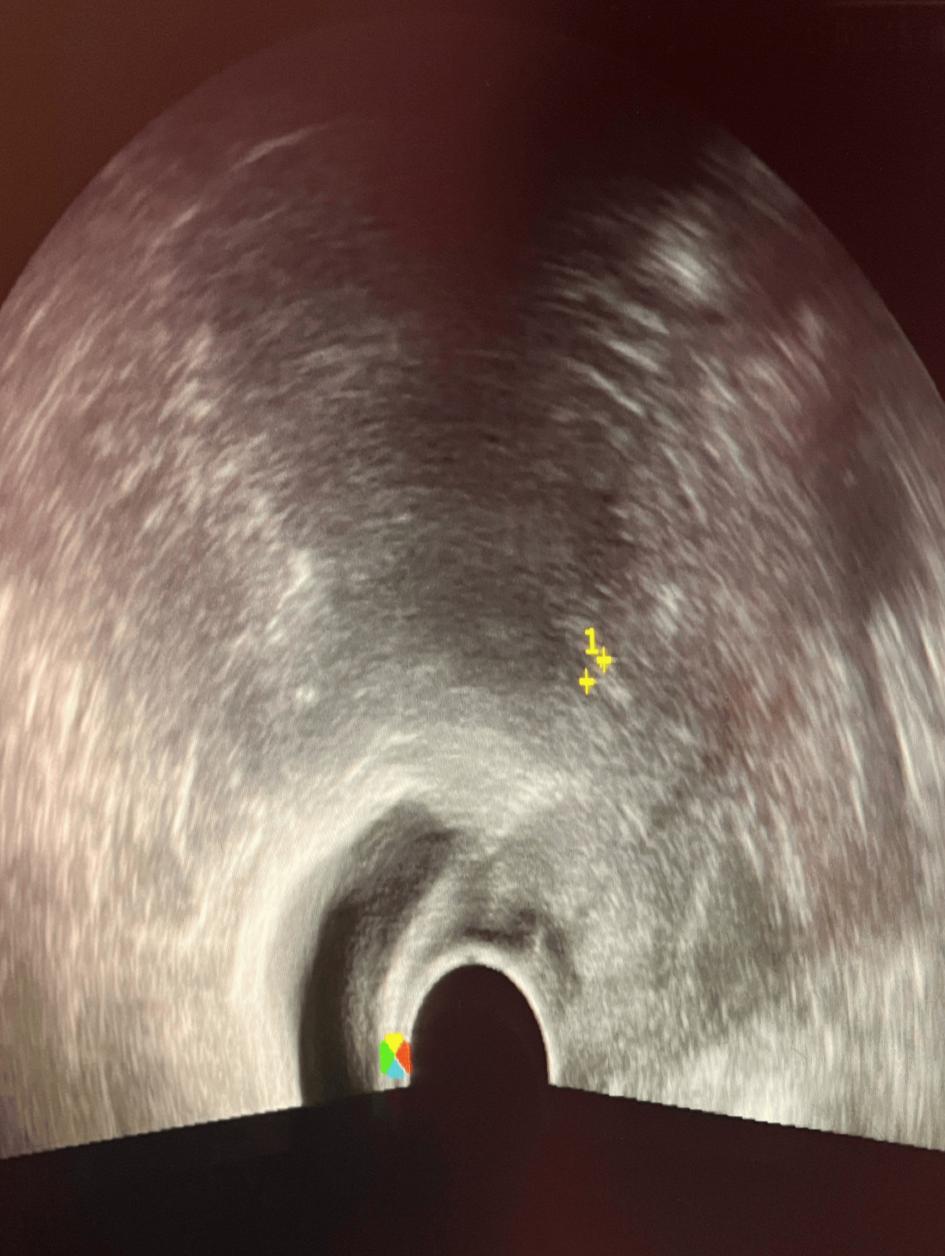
Follow-up transvaginal ultrasound image Post-removal sonogram demonstrates a regular, thin endometrial lining with homogeneous echotexture, measuring approximately 3 mm in thickness. No residual intrauterine device fragments or endometrial abnormalities are observed.
